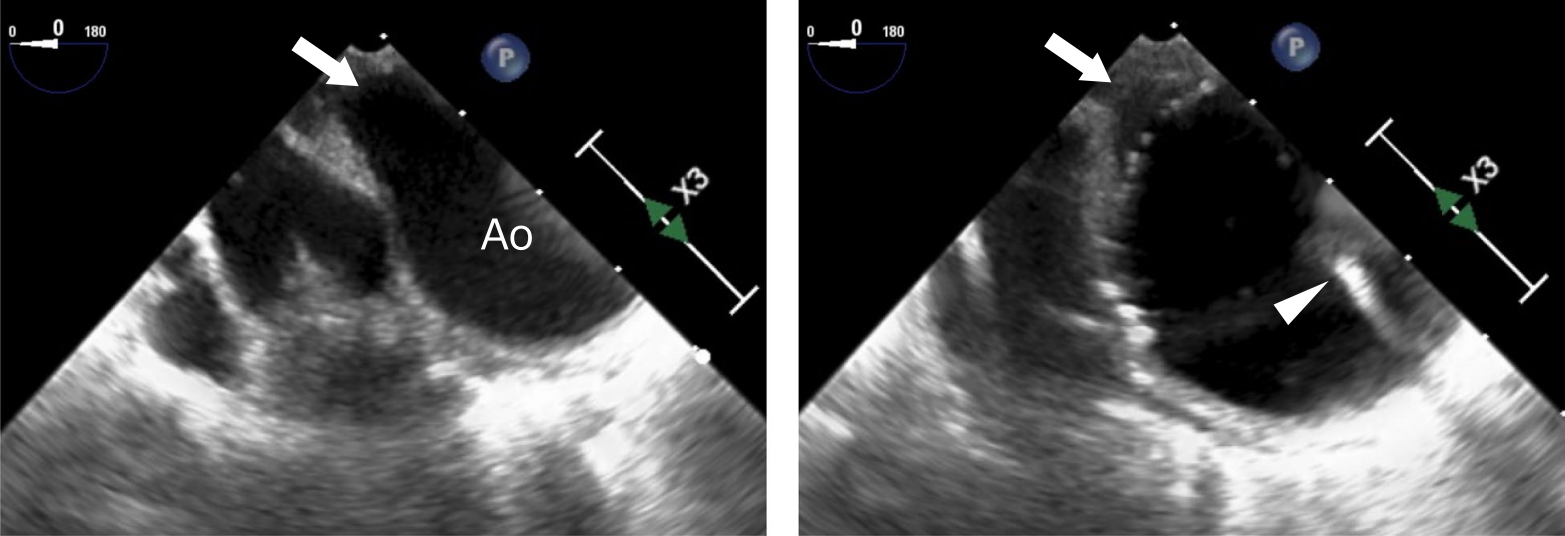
Transesophageal echocardiography revealed the MAPCA (arrow) originating from the descending aorta. The inflated intra-aortic balloon catheter (arrowhead) blocked the pulmonary blood flow through the MAPCA. Ao, aorta
